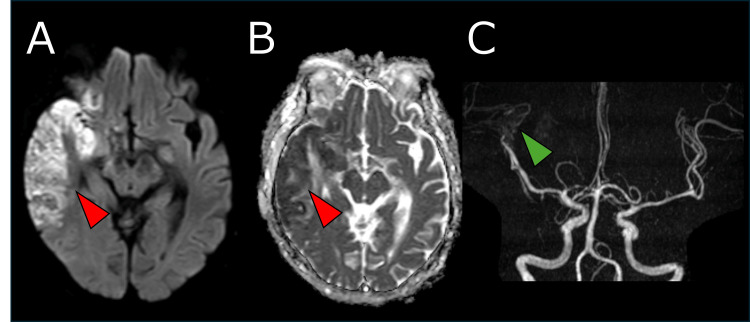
Brain MRI on Day 9. Follow-up diffusion-weighted imaging (A) showed extensive high signal intensity in a widespread area of the right MCA territory, with corresponding low values on apparent diffusion coefficient mapping (B) (red arrowheads). MRA demonstrated poor visualization of the M2 segment of the MCA (C; green arrowhead). MCA: middle cerebral artery, MRA: magnetic resonance angiography, MRI: magnetic resonance imaging.
